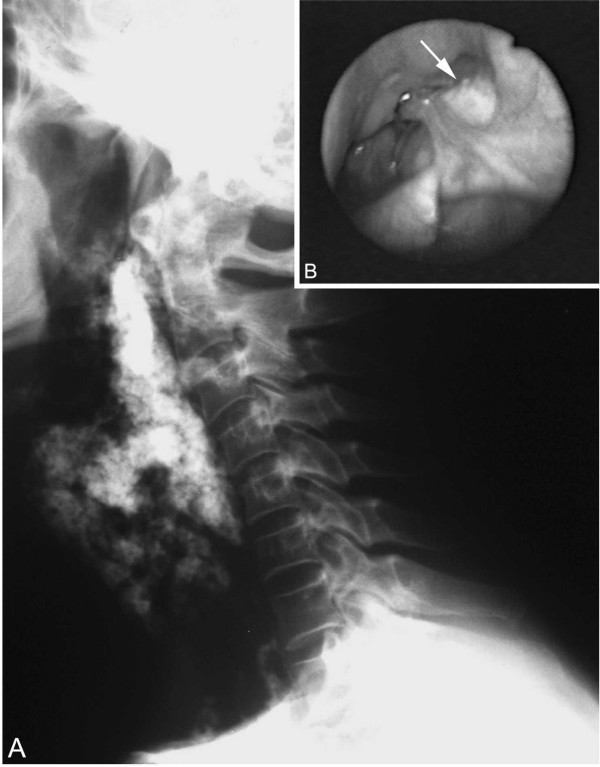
A. Neck radiograph evidences grossly calcified areas of soft tissue in the topography of the oropharynx. B. Direct laryngoscopic examination shows exophytic calcic deposits in the left pyriform sinus (arrow).
